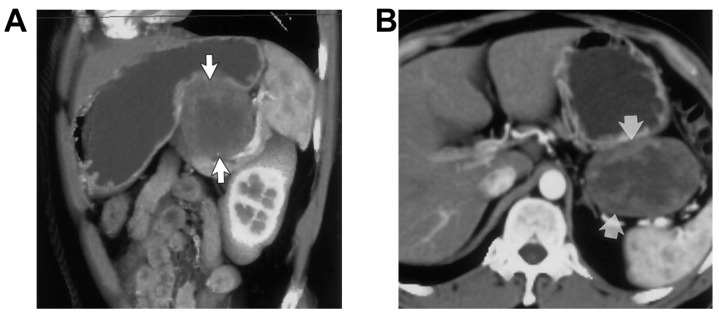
Sagittal (A) and axial (B) oblique contrast-enhanced 3D volume-rendered CT scans revealed a round exophytic mass in the stomach, 5-cm exophytic mass (arrows) that arises from the stomach, which proved to be a benign GIST during surgery. Reprinted with the permission of [183].
